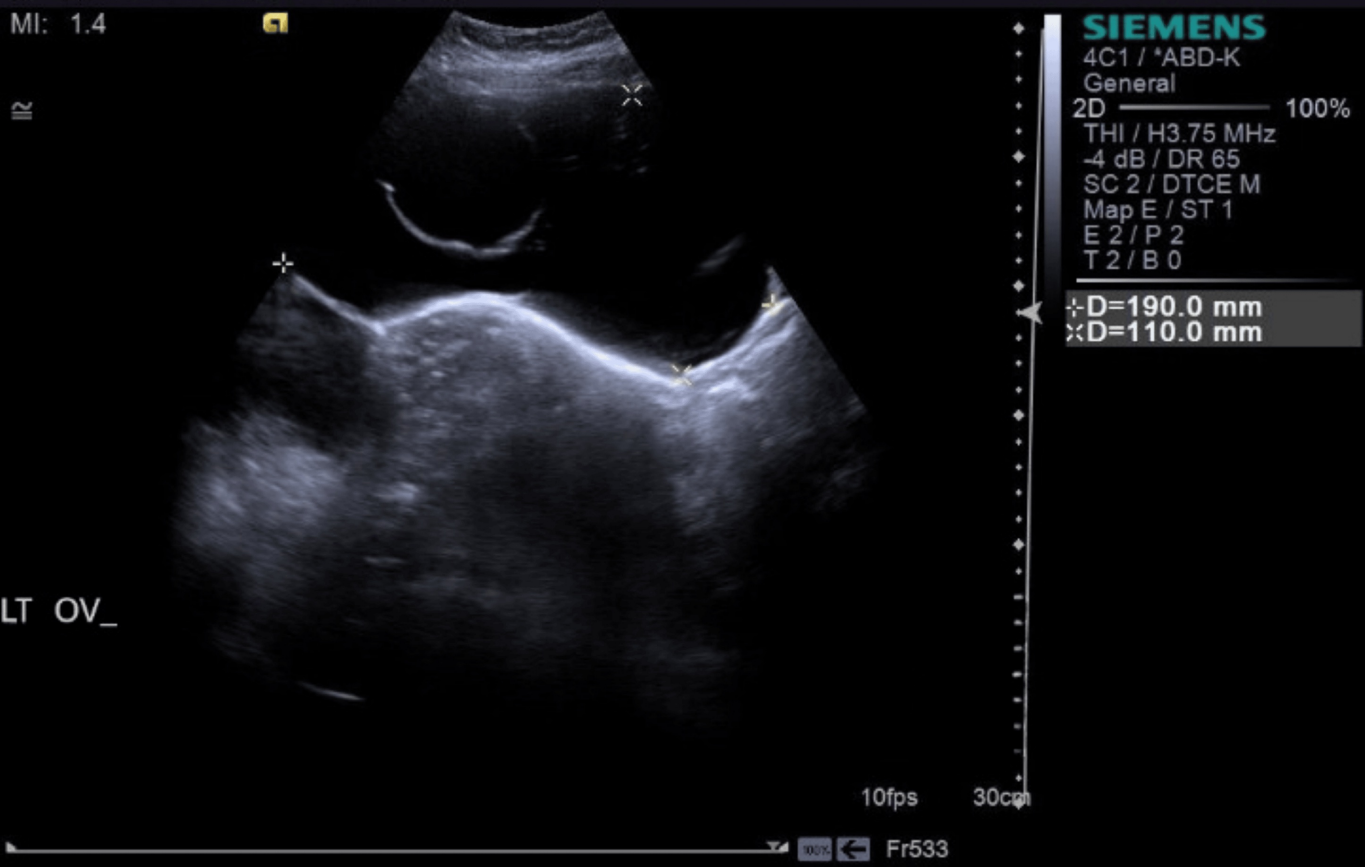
Pelvic ultrasonography showing a left ovary cyst with dimension 190.0 mm × 110.0 mm
